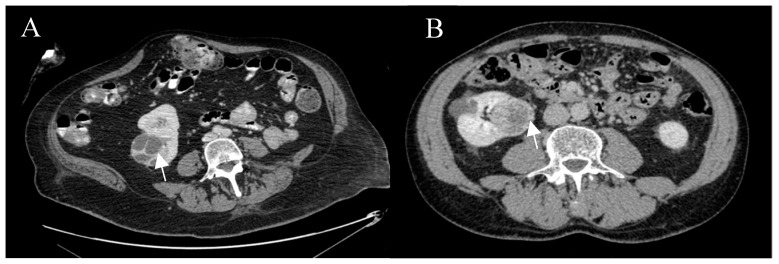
Post-contrast axial CT images of complex right renal cysts. (A) Bosniak IV cyst, conventional clear cell, pt3a, grade 2, resected by radical nephrectomy. (B) Bosniak IV cyst, oncocytoma, resected by partial nephrectomy.
